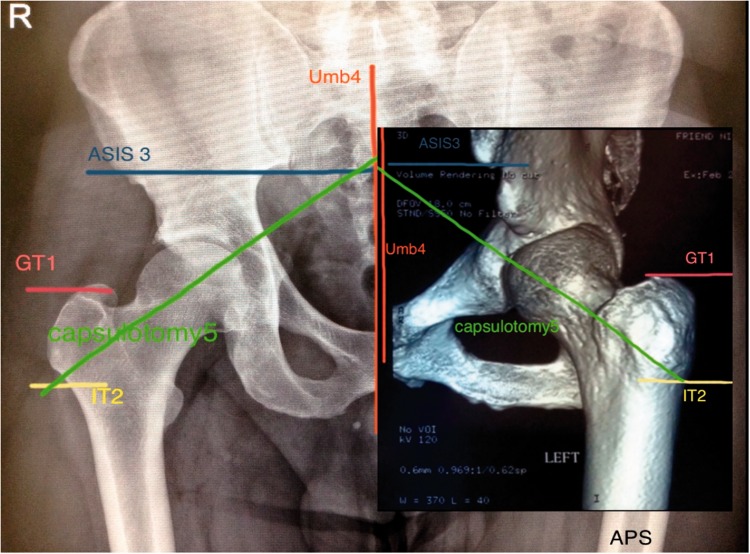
Radiographic landmarks plotted preoperatively in an AP of the Pelvis and also reproduced in an oblique view of a 3D CT scan.
